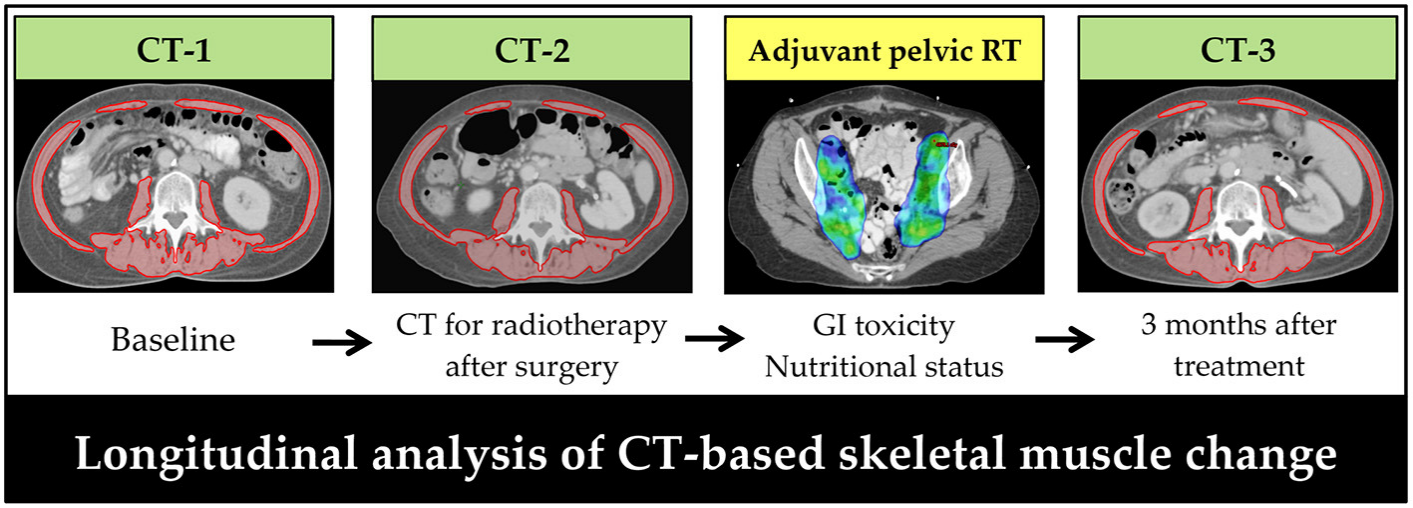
Timeline of computed tomography scans for patients with early-stage cervical cancer receiving surgery and post-operative pelvic radiotherapy. Skeletal muscle was assessed on a transversal computed tomography slice at the level of L3. Red: skeletal muscle area. CT, computed tomography; RT, radiotherapy.
